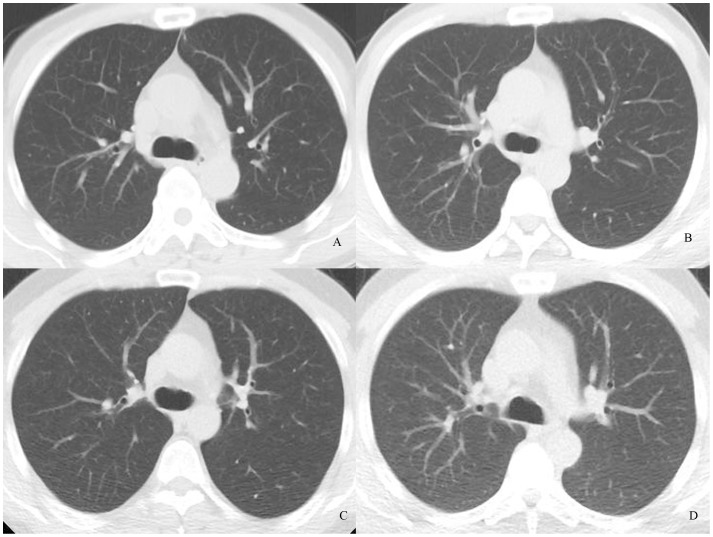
Transverse chest CT images of 4 patients who were almost the same height and weight. Images were reconstructed with 30% ASIR. Images are (A) NI = 15, objective image noise 10.95; (B) NI = 20, objective image noise 18.28, (C) NI = 30, objective image noise 28.5, and (D) NI = 40, objective image noise 41.52. The visibility of the small structure (arrow) in D was around average.
